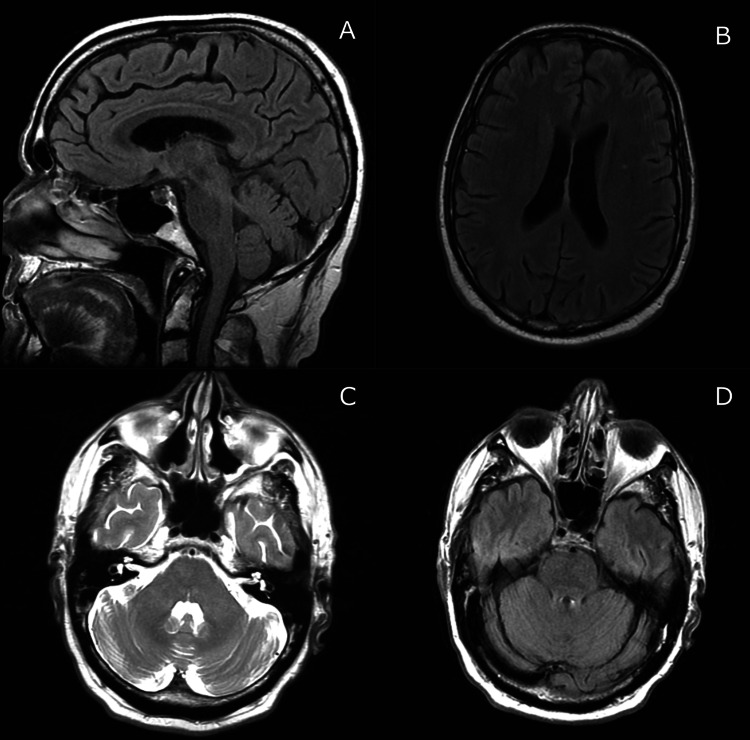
MRI of the brain 12 months after initiation of chemotherapy revealed no evidence of central nervous system lymphoma and resolution of the previously questioned cerebellar demyelination. A: Sagittal T1-weighted image; B: Coronal T1-weighted image; C: Coronal T2-weighted image; D: FLAIR image.
